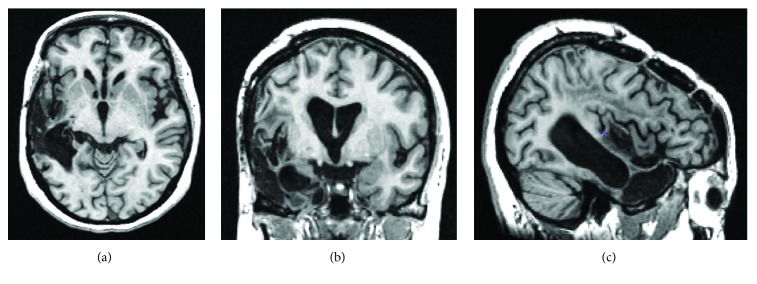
MR T1-weighted image of the patient's brain in (a) axial, (b) coronal, and (c) sagittal views. The patient presents with an extensive area of encephalomalacia within the left cerebral hemisphere involving the temporal and frontoparietal lobes with volume loss.
